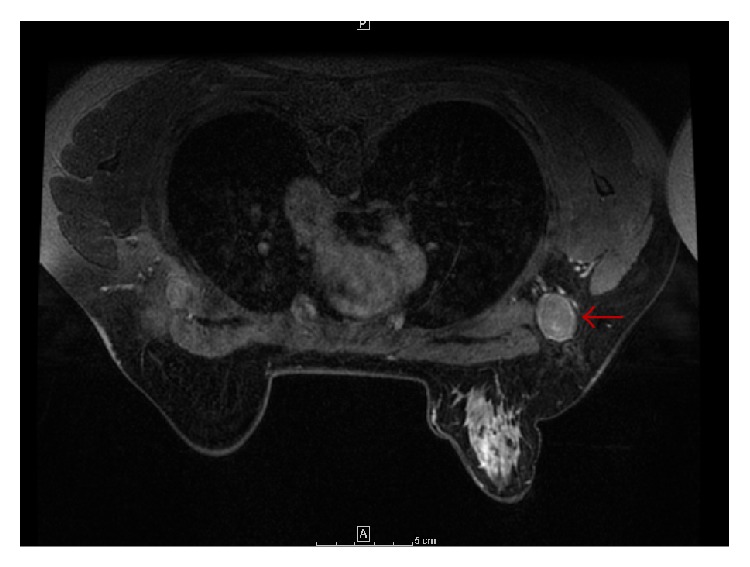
MRI breast: axial T1 image with contrast demonstrating normal breast tissue with right axillary lymphadenopathy (red arrow).
